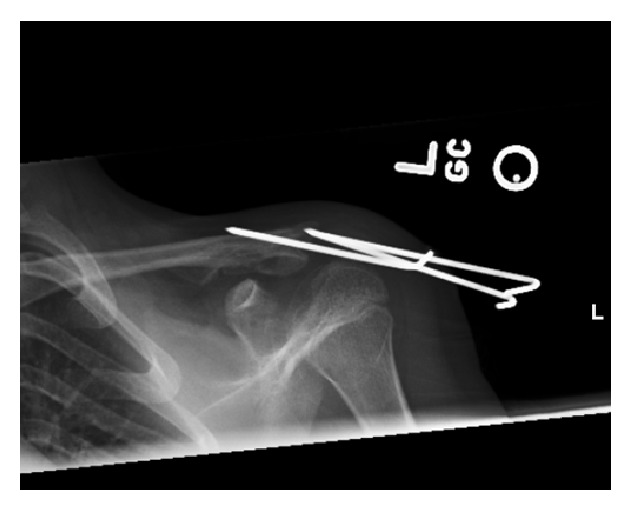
Radiographs of the left shoulder showing reduction of the clavicle fracture and fixation by three wires.
